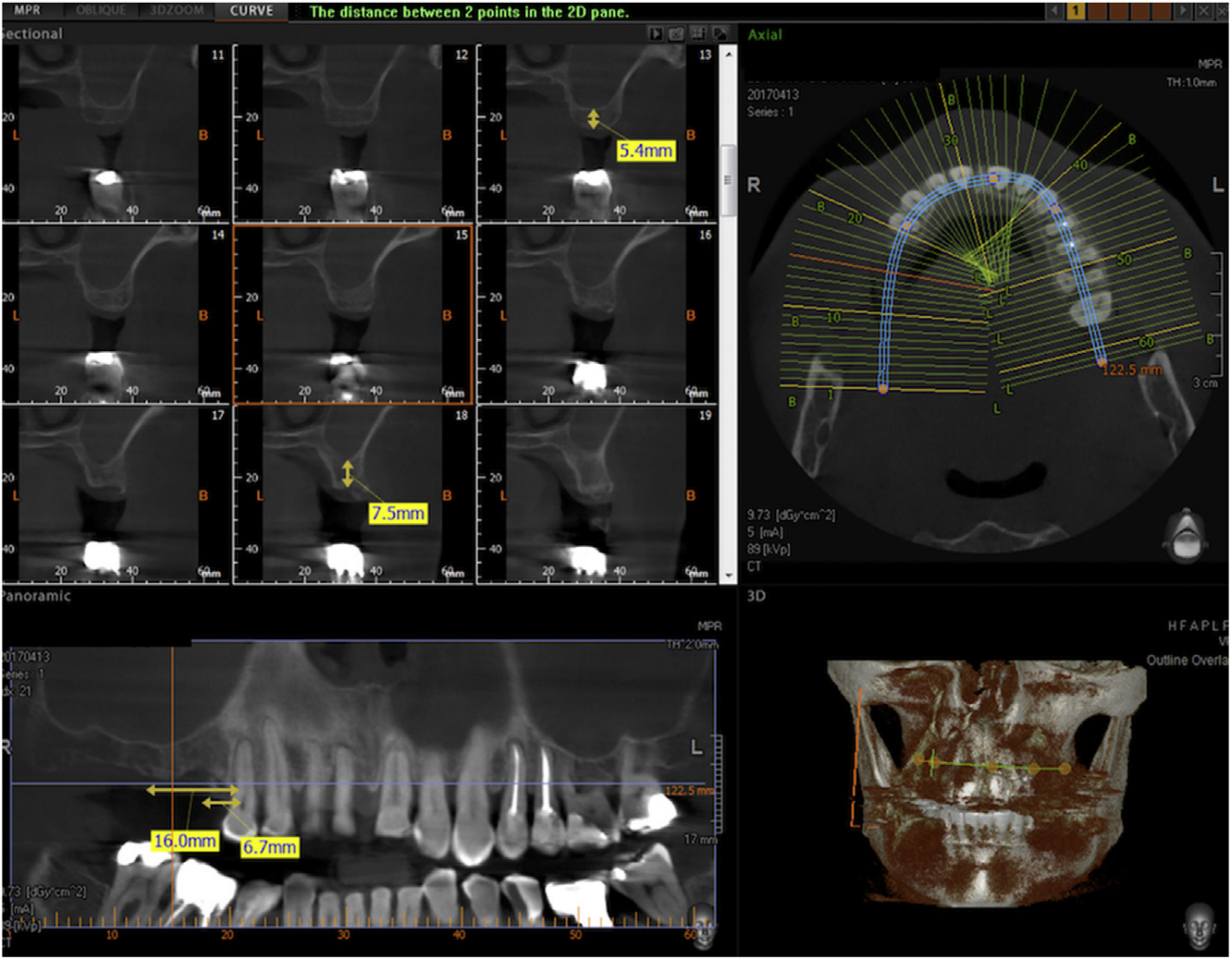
Preoperative CBCT of a case selected for the Flapless LMSF Technique. The treatment plans is to insert 2 implants in the upper right sextant in the positions of the second bicuspid and the first molar. The alveolar bone height is 5.4 mm in the molar site and 7.5 mm in the bicuspid site. The width is sufficient for implant placement without expansion. The CBCT shows signs of chronic sinusitis (asymptomatic). The middle meatus is unobstructed.
